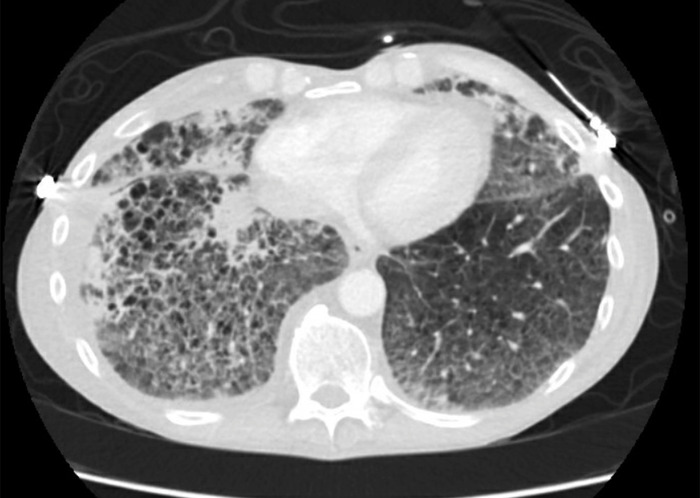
Admission chest computed tomography, transverse view of lower lobes, shows new onset diffuse interstitial pulmonary ground-glass airspace opacities.
